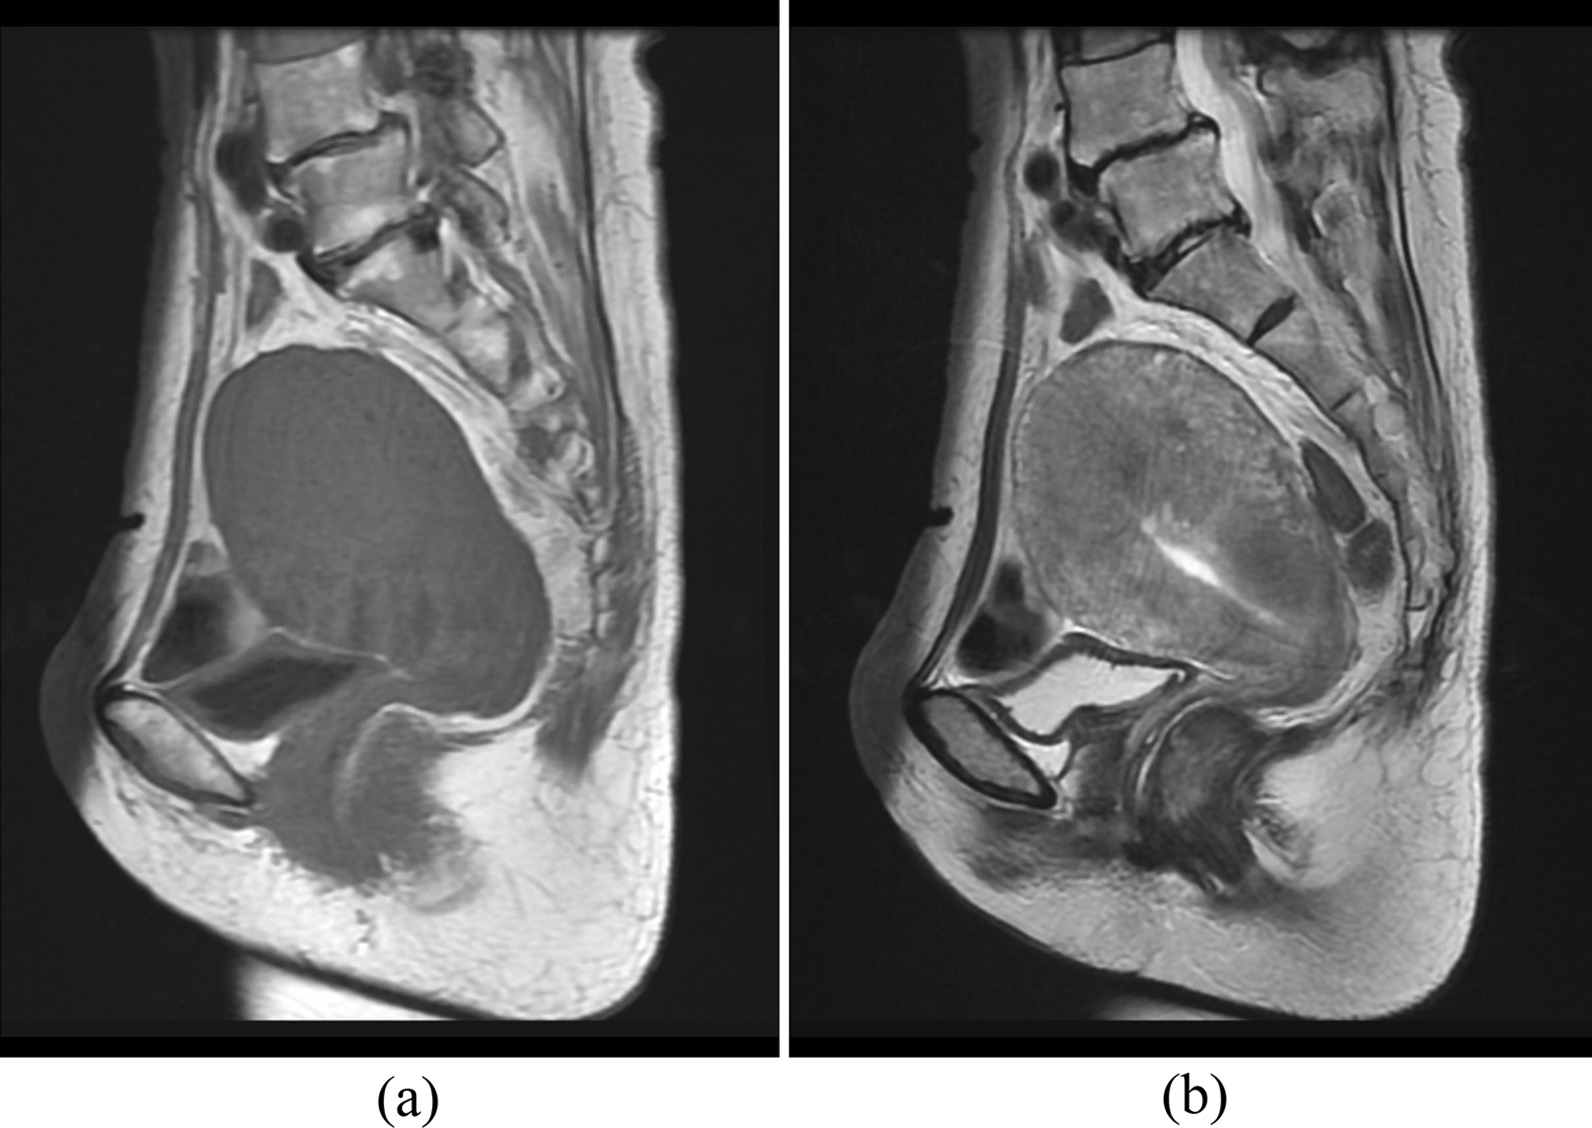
Magnetic resonance imaging demonstrated an enlarged uterus with thickening of the entire uterine wall, suggesting adenomyosis. Both T1-weighted (a) and T2-weighted (b) imaging revealed no uterine nodules
